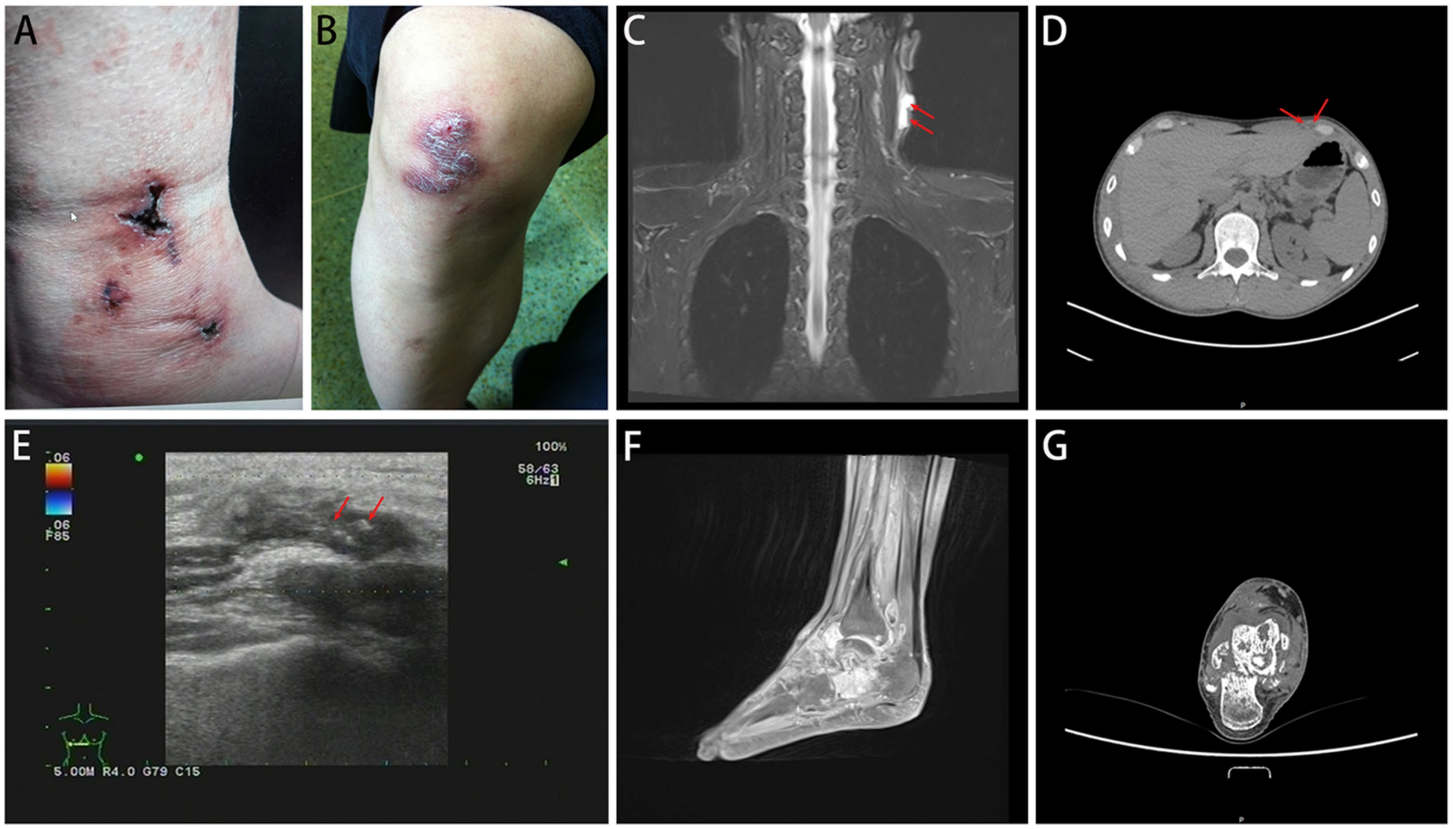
Imaging examination of CTB. CTB appears as ulcers (A) or nodules (B). (C,D) CT scans indicated local soft tissue swelling and flaky low-density shadows. (E) Ultrasound typically showed irregular hypoechoic areas under the skin. (F,G) MRI revealed multiple changes in bone and soft tissue.
